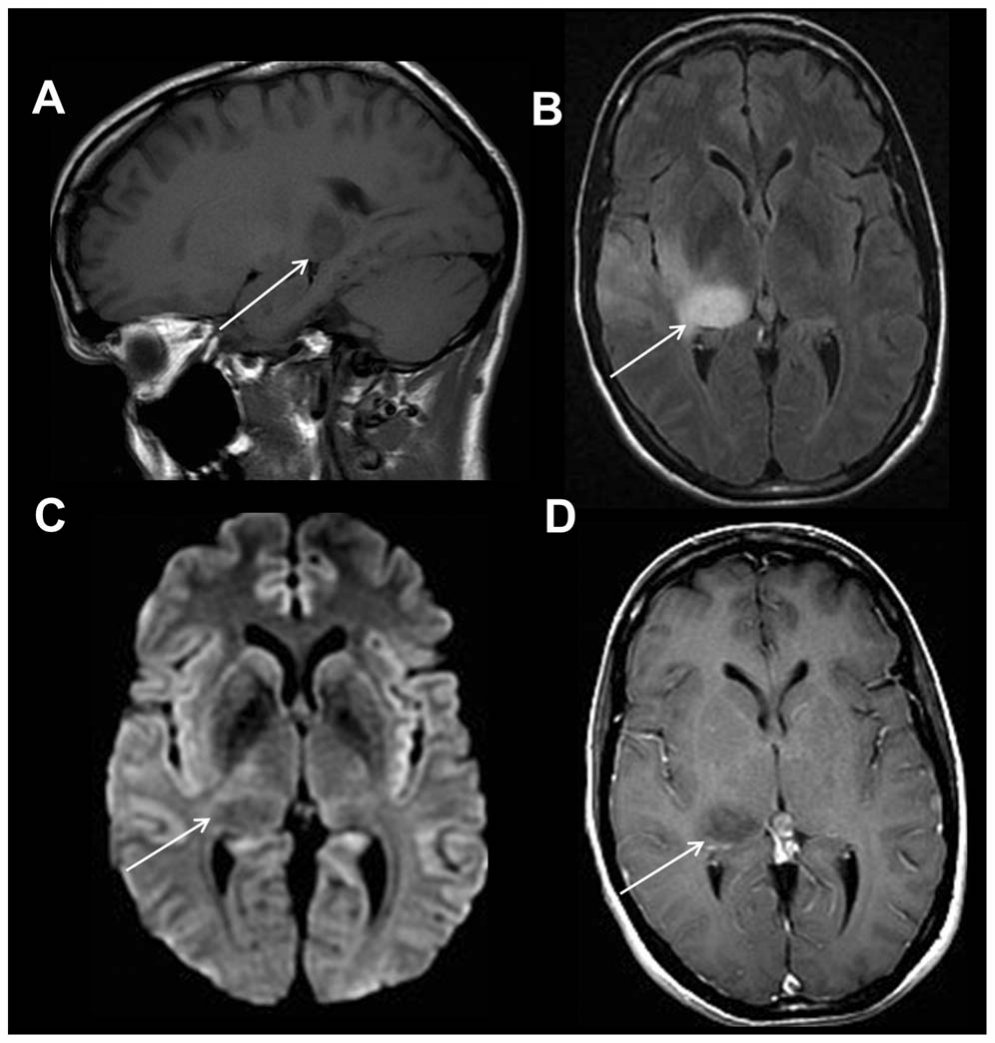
Right thalamic neoplasm identified in a 48 year old female with seizures. Limited pre-contrast set of imaging sequences are sufficient to clearly identify an expansile lesion within the pulvinar of the right thalamus; a finding requiring immediate communication for action (Level I) (white arrows). It is hypointense on sagittal T1-weighted image (A) and has associated hyperintensity on axial T2-weighted FLAIR extending into the adjacent insular-opercular region (B). Even within the confines of the limited set of sequences, neoplastic differential considerations are still able to be favored over acute ischemia as no evidence of restricted diffusion is seen on diffusion-weighted imaging (DWI) sequences; specifically low signal on DWI trace images (C) and elevated diffusivity on Apparent-Diffusion-Coefficient images (not shown). Post-contrast imaging, not initially available to the readers within the limited set of review sequences, demonstrated no abnormal enhancement (D).
